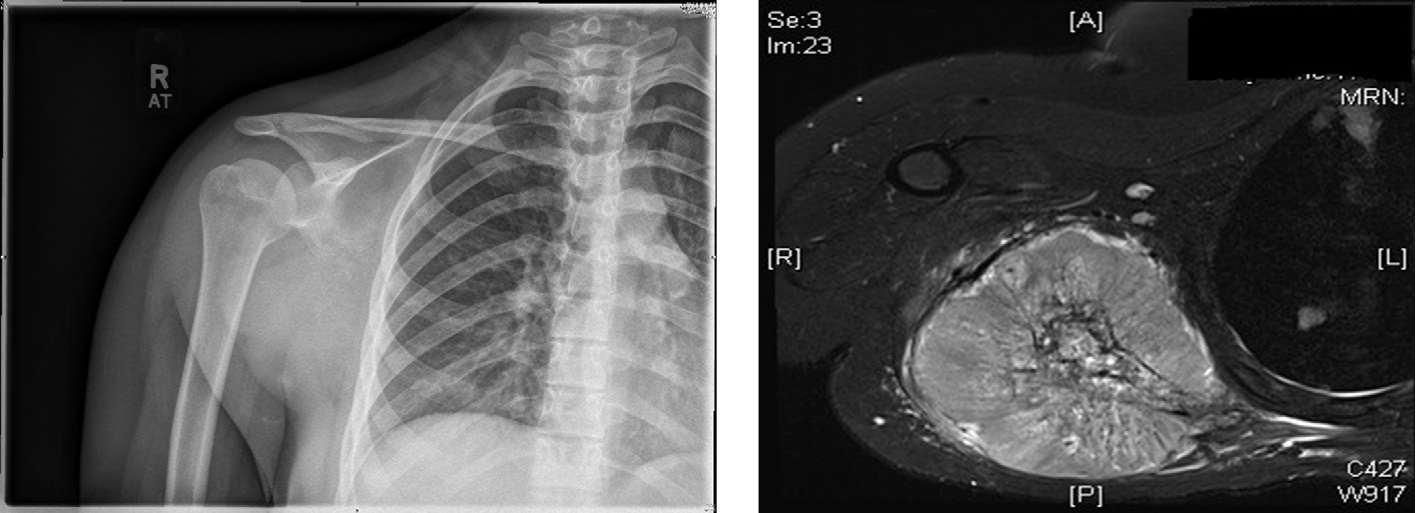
Plain radiograph demonstrating a destructive lesion of the right scapula confirmed with axial view of MRI scan showing a large soft tissue component to the scapular lesions consistent with Ewing’s sarcoma.
